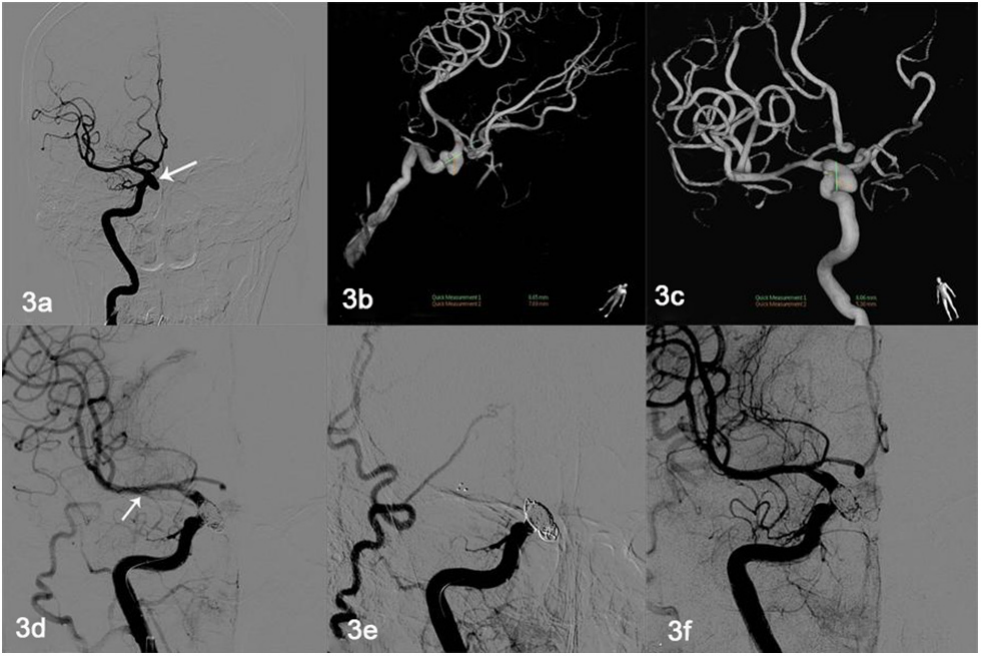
Thromboses throughout the stent in one patient. (a–c) Figure depicts a 46-year-old man with right carotid-ophthalmic wide-necked ruptured aneurysm (8.69 mm × 7.69 mm). (d) Solitaire AB stent (6 mm × 30 mm) assisting embolization (white arrow); there was parent artery patency. (e) Acute stent thrombosis 3 h later; and 0.3 million U urokinase was administered through the microcatheter. (f) Repeat radiography revealing expedited blood flow through the stent.
